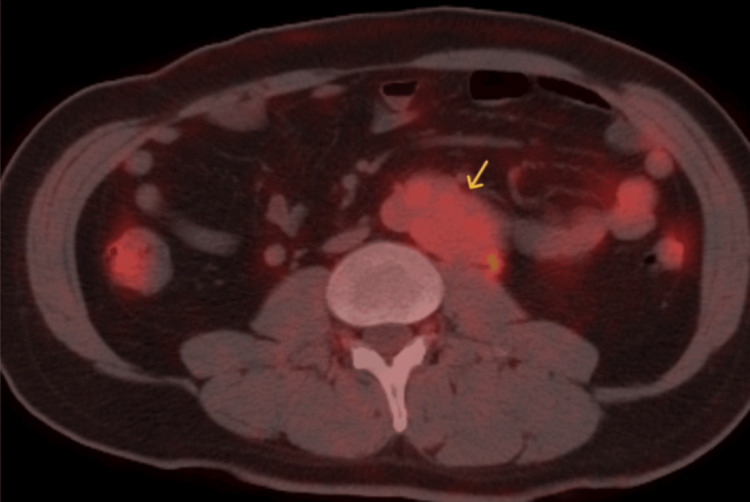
PET-CT scan There is a low-intensity tumor (arrow) with an SUVmax of approximately 2.5, with no discernible signs suggestive of distant metastasis. The tumor is in contact with the left ureter PET, positron emission tomography; CT, computed tomography; SUVmax, maximum standardized uptake value
